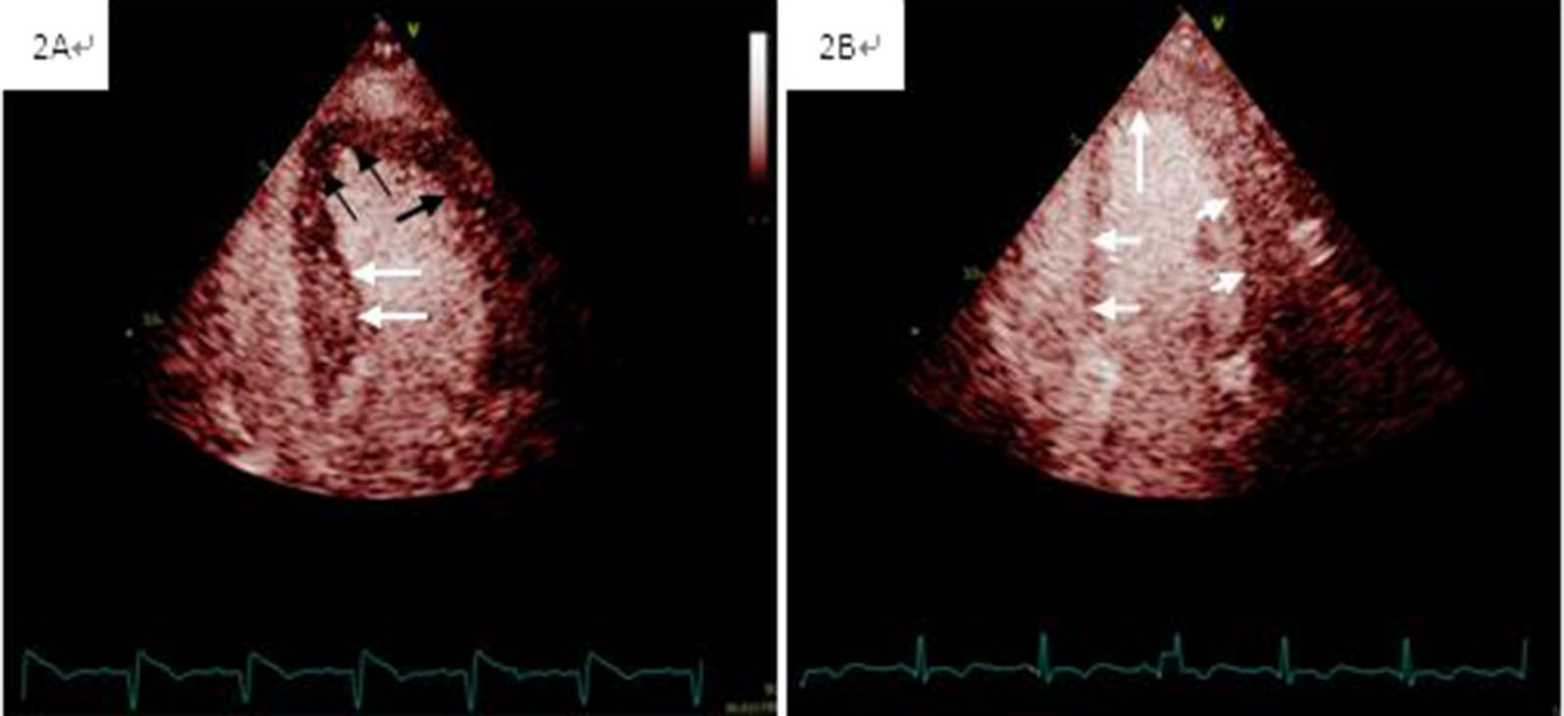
Examples of abnormal microvascular perfusion and normal microvascular perfusion. Demonstration of abnormal (A) and normal (B) myocardial contrast replenishment on real-time myocardial contrast echocardiograph. Black arrows: myocardial perfusion defect; white arrow: normal myocardial perfusion
